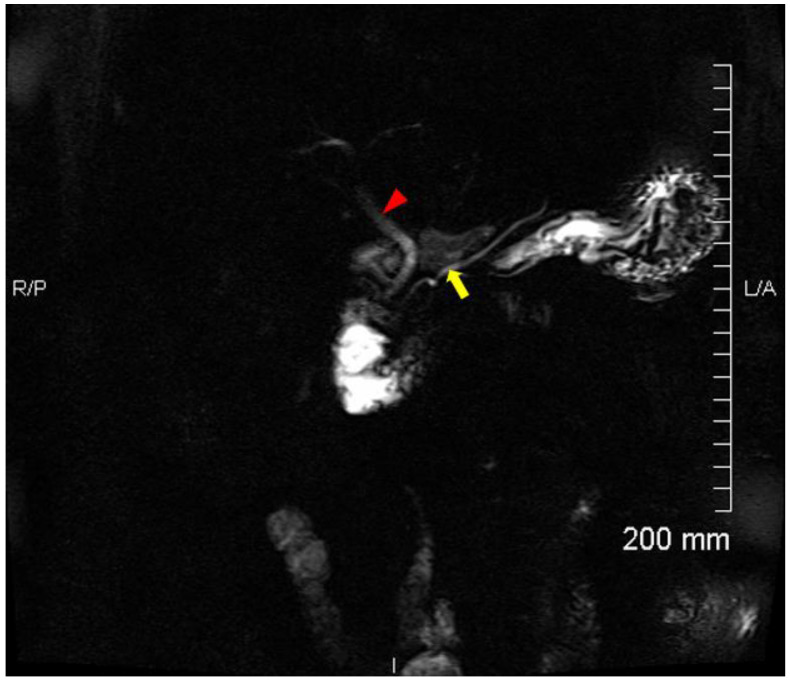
A follow-up MRCP 9 days after the ^18^F-FDG PET shows no evidence of acute cholangitis or acute pancreatitis. Coronal T2 weighted MIP imaging (SSTSE, slice thickness 30 mm) shows the CBD (arrow head) and main pancreatic duct (arrow). No lesions or abnormal findings within the CBD. The size of the CBD measures 6 mm.
